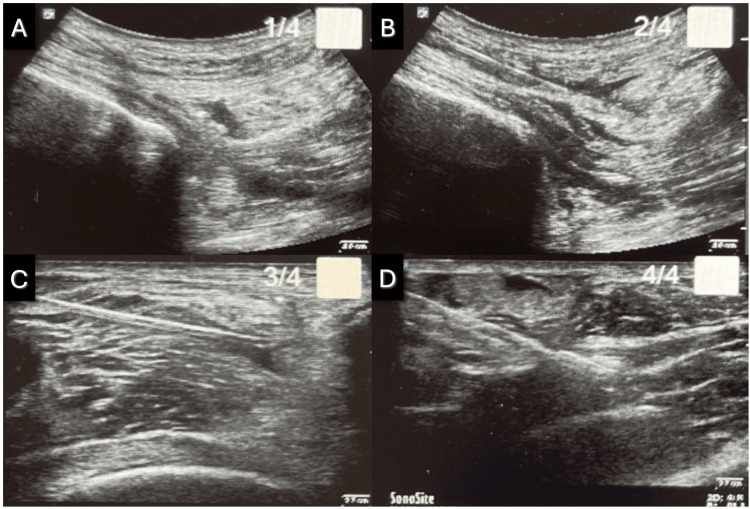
Ultrasound-guided nerve block: (A) left transgluteal sciatic nerve block, (B) left lateral femoral cutaneous nerve block, (C) left femoral nerve block, (D) left obturator nerve block.
